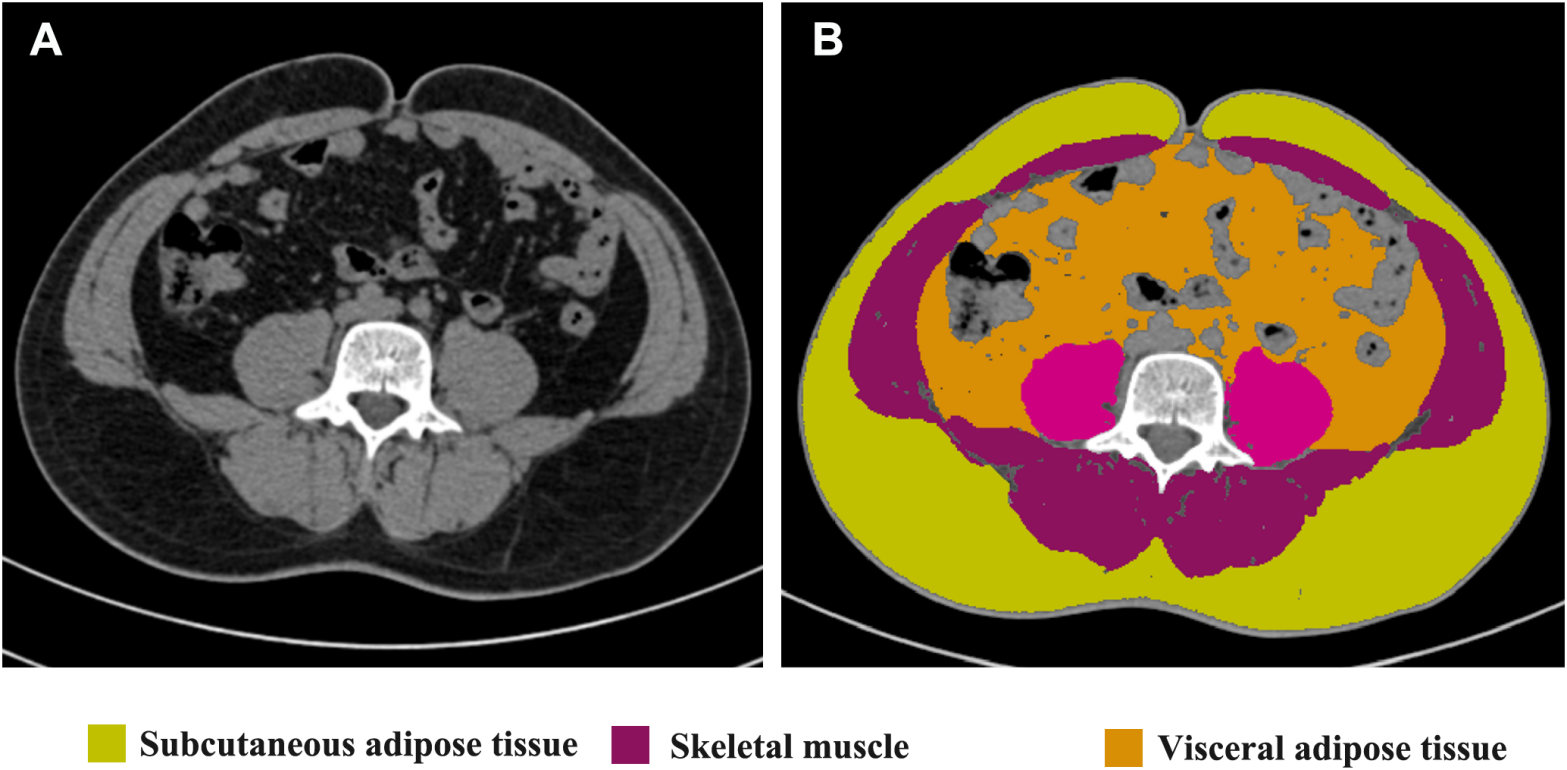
Radiological measurements on the CT scans. Unprocessed CT slice at the level of the umbilicus performed preoperatively is shown in (A). Body metrics evaluation performed using slice‐O‐matic image analysis software is shown in (B). Extraperitoneal skeletal muscles (purple), subcutaneous adipose tissue (yellow) and visceral adipose tissue (brown) are seen.
